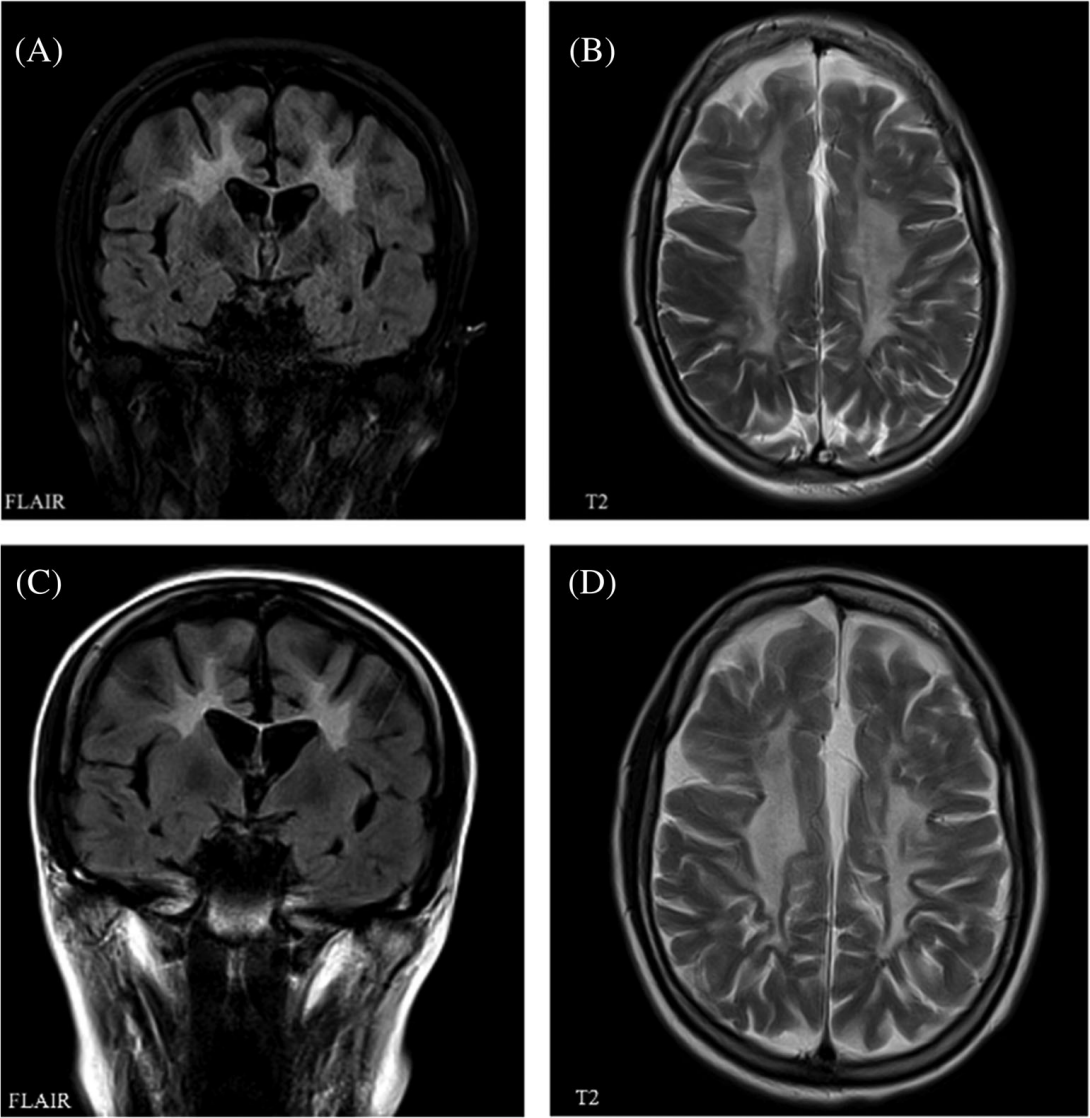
Brain MRI of patient A before and 36 months after transplantation. A (coronal section, FLAIR) and B (axial section, T2 weighting) show MRI performed 4 months before transplantation (age 20 years and 11 months), both showing widespread, symmetrical, and confluent supratentorial white matter changes typical of MLD and a MLD‐MRI score of 21/34. The typical tigroid pattern can be seen in B. C (coronal section, FLAIR) and D (axial section T2 weighting) show MRI performed 36 months posttransplantation (age 22 years and 9 months) with unchanged extensive white matter pathology, MLD‐MRI score unchanged of 21/34
